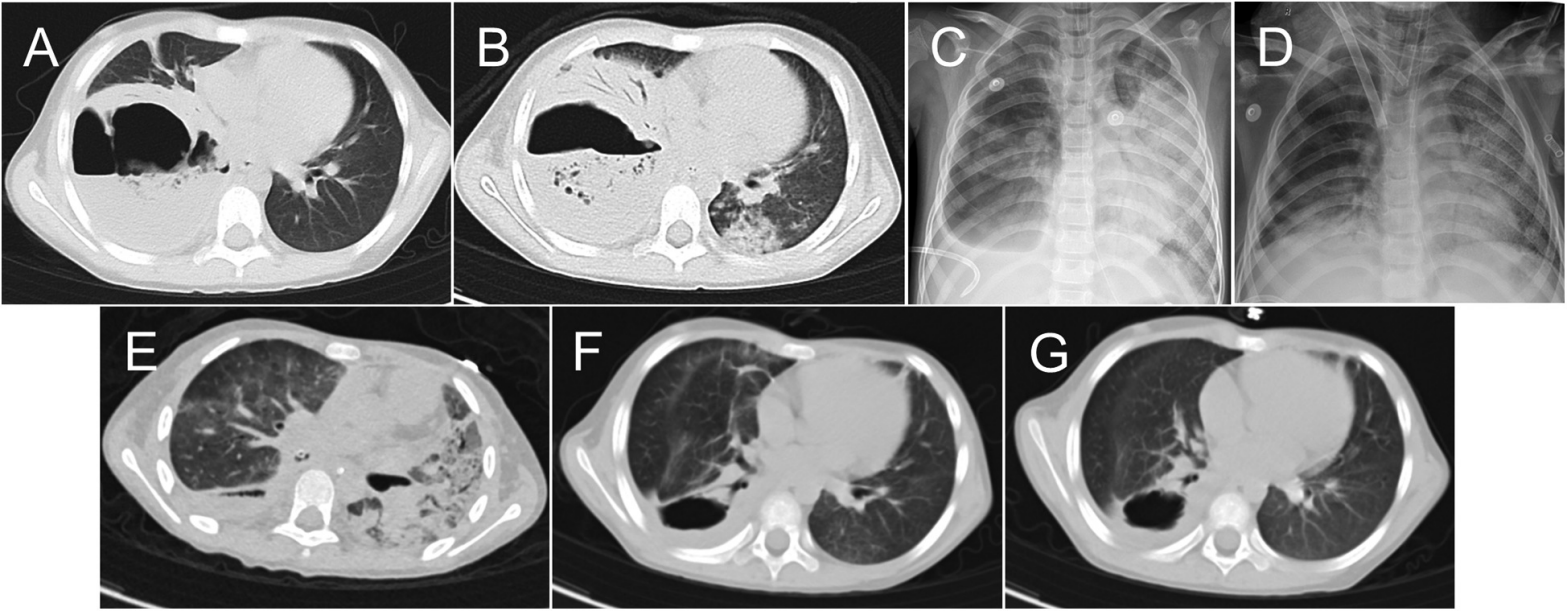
Chest images obtained during and after the patient's hospital stay. Chest computed tomography (CT) images obtained on days 1 (A) and 6 (B) of hospitalization. Chest x-rays obtained before extracorporeal membrane oxygenation initiation (C) and weaning (D) Chest CT images obtained on days 36 (E) and 65 (F) of hospitalization and 1 month after discharge (G).
